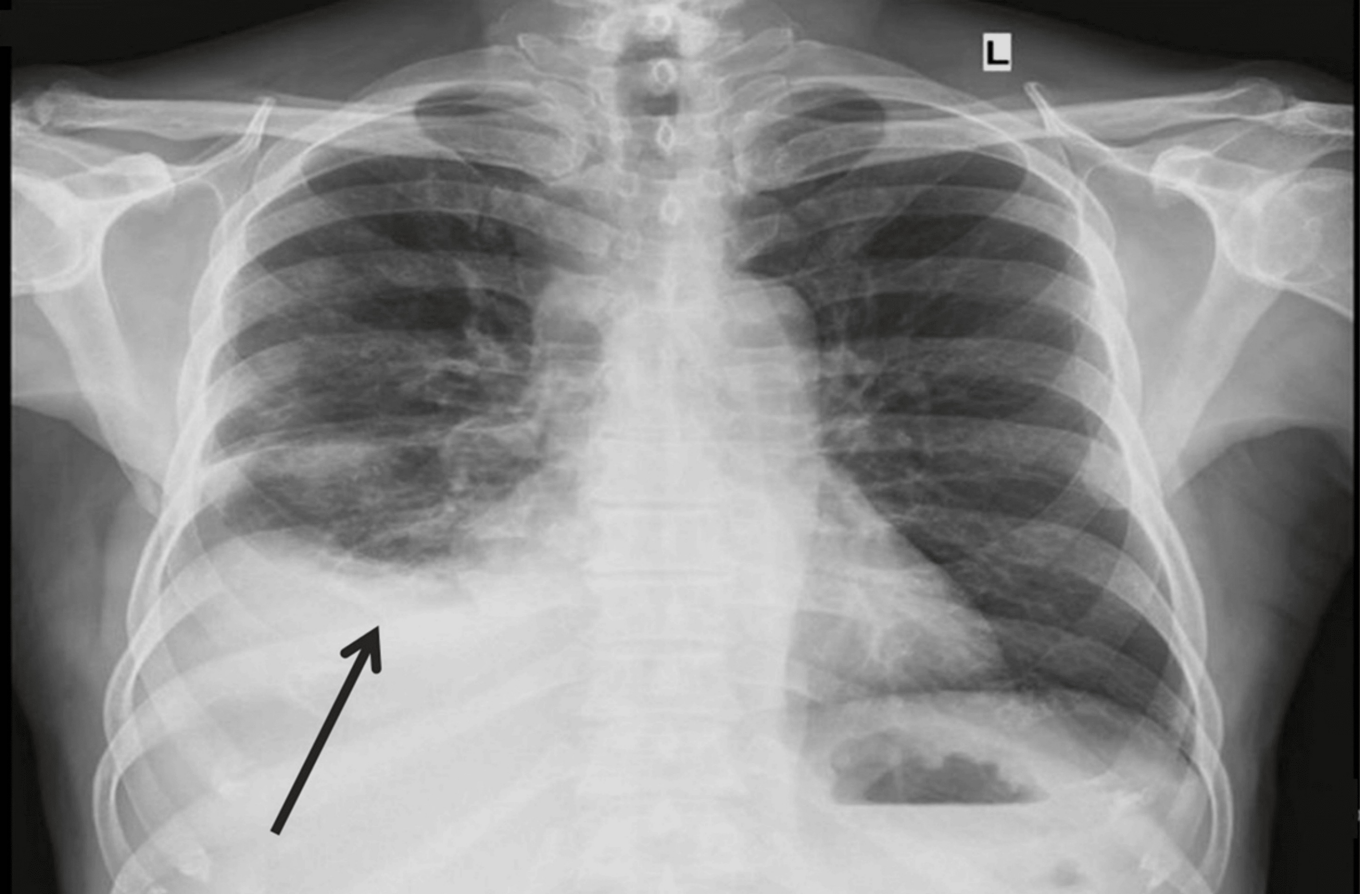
Chest radiograph showing right pleural effusion with no mediastinal shift
